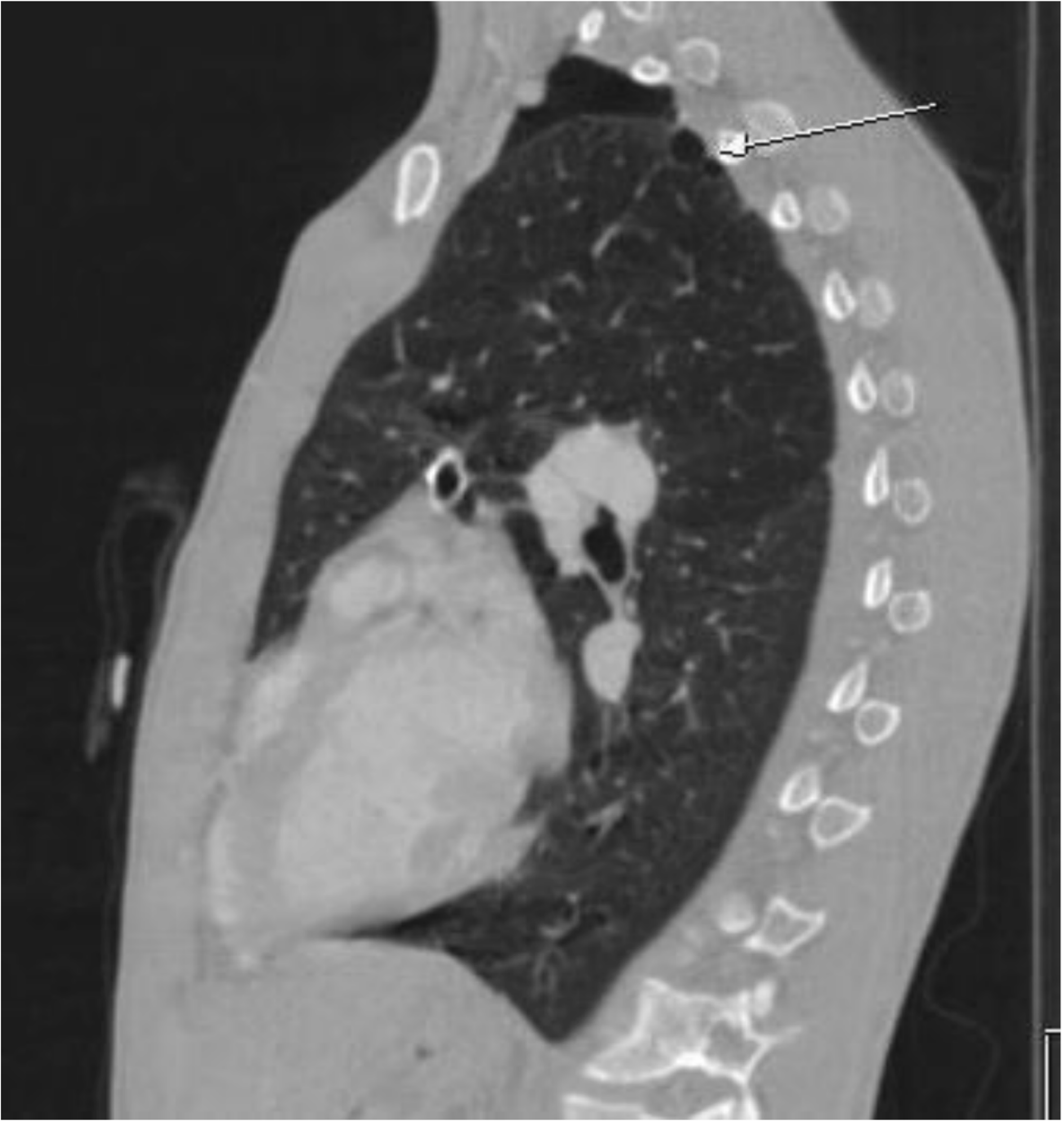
CT scan angiography in our patient with SJMS, showing remaining pneumothorax with presence of apical emphysematous bulla in the left lung (arrow). Note hyperlucency of the upper pulmonary lobe in comparison to the lower.
